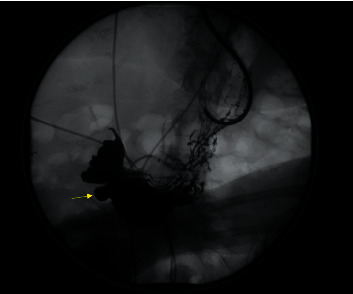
Upper gastrointestinal contrast radiographic study. The patient was discovered to have a distal antral diverticulum of the under surface of the gastric body (arrow).
